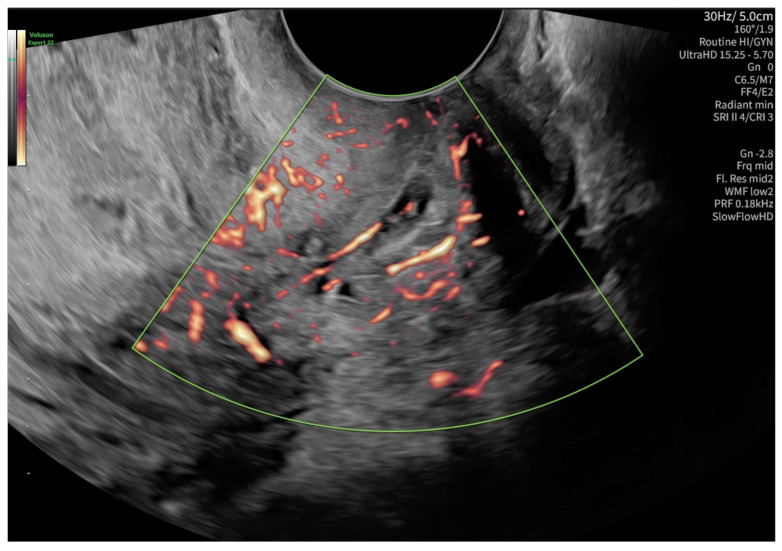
Blood flow around the gestational sac in a color Doppler examination.
